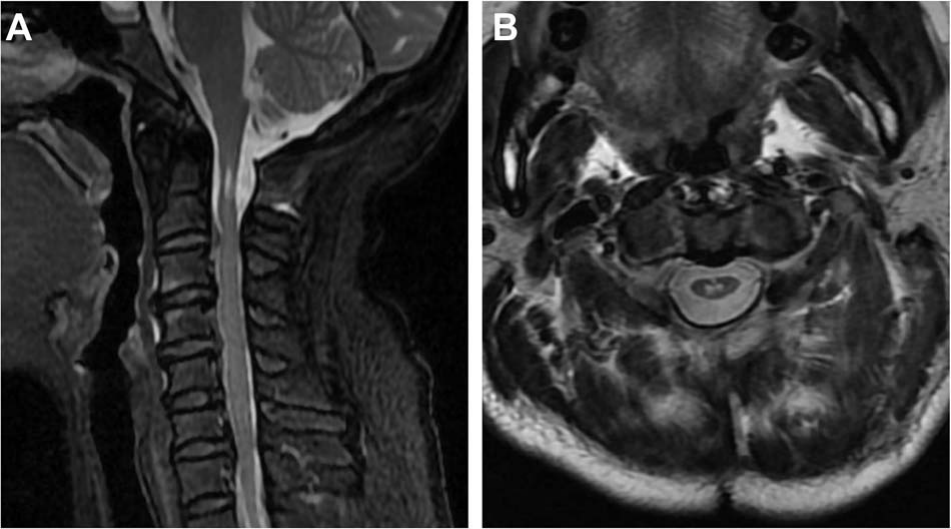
Two-year postoperative MRI results. (A) Sagittal T2-weighted MRI. (B) Axial T2-weighted MRI.
